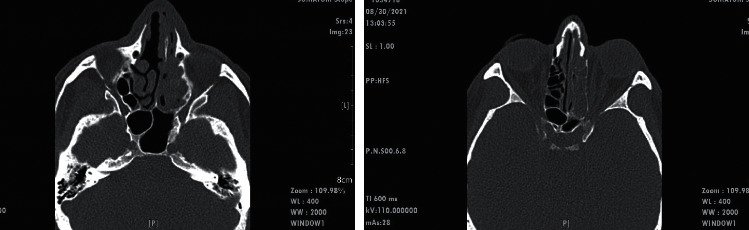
CT scan of sinuses (axial and coronal) revealed nasal septum is deviated to the left side. Opacity is seen in the left maxillary sinus and ethmoidal sinuses.
